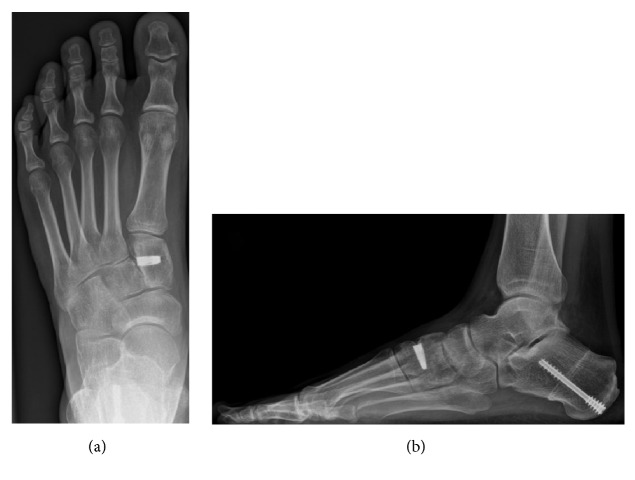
Postoperative (titanium wedge) anterior-posterior (a) and lateral (b) weight-bearing radiographs.
